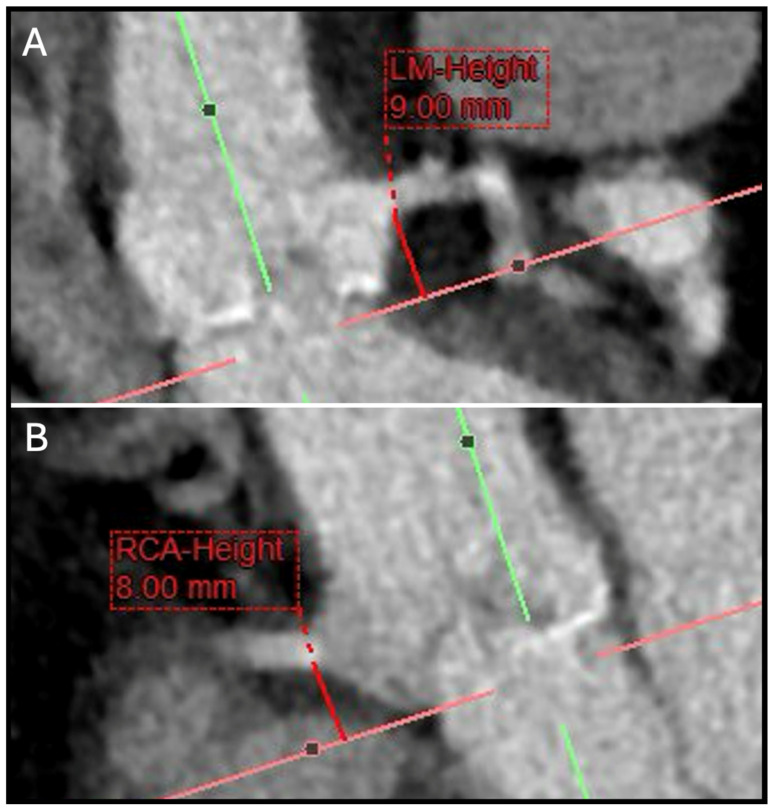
Pre-TAVR CT work-up with two examples of coronary ostia height (A) LM-Height (B) RCA-Height. The coronary height should by assessed by measuring the distance from the aortic annulus plane to the lower-level margin of the coronary ostia. CT: computed tomography; LM: left main; RCA: right coronary artery;TAVR: transcatheter aortic valve replacement.
